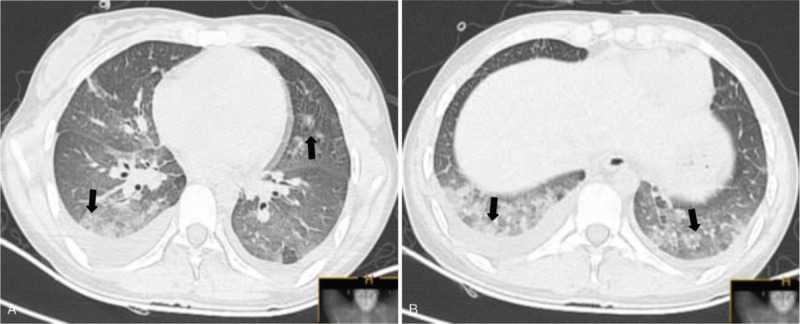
(A, B) Chest computed tomography scan on admission revealed bilateral ground glass opacities, patchy nodular, and pleural effusions.
